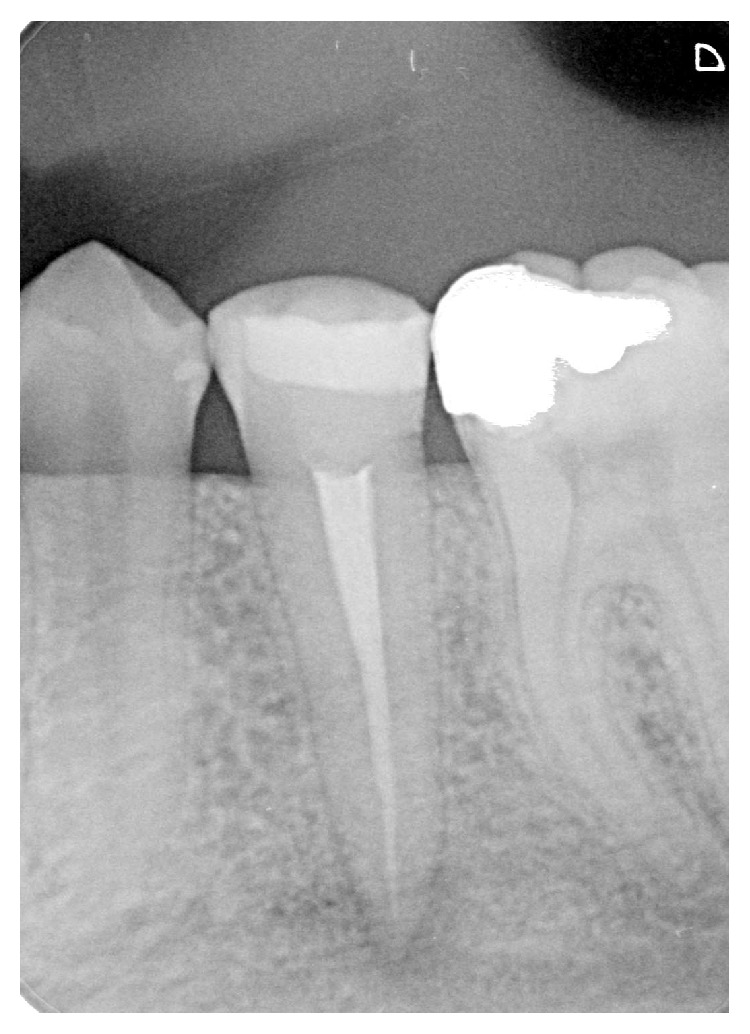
Periapical radiograph of mandibular left second premolar taken after root canal treatment.
